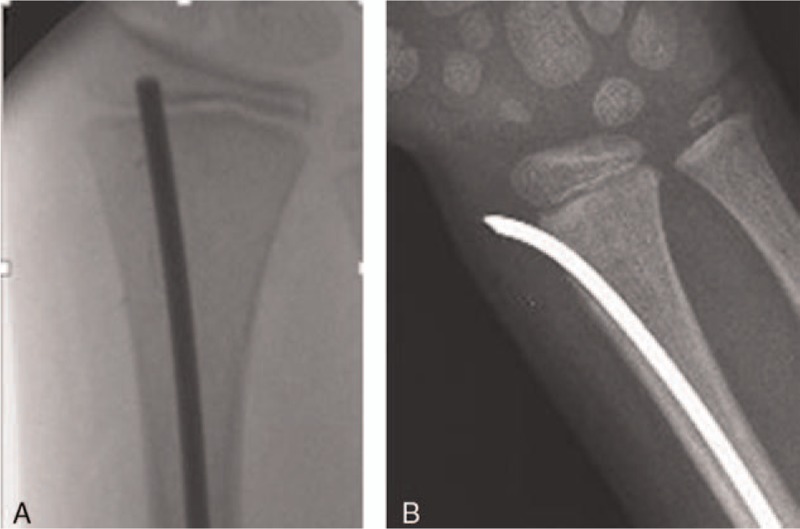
(A) X-ray of dorsal entry elastic nailing. (B) X-ray of radial entry elastic nailing.
